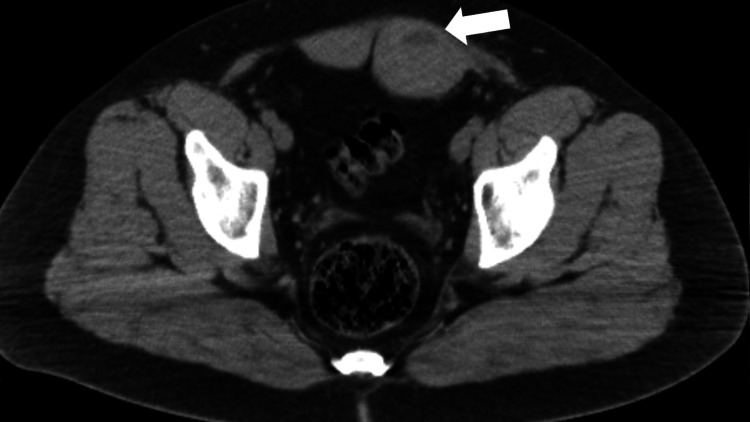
RSH located in the left rectus muscle. Axial CT images (Patient 11)
